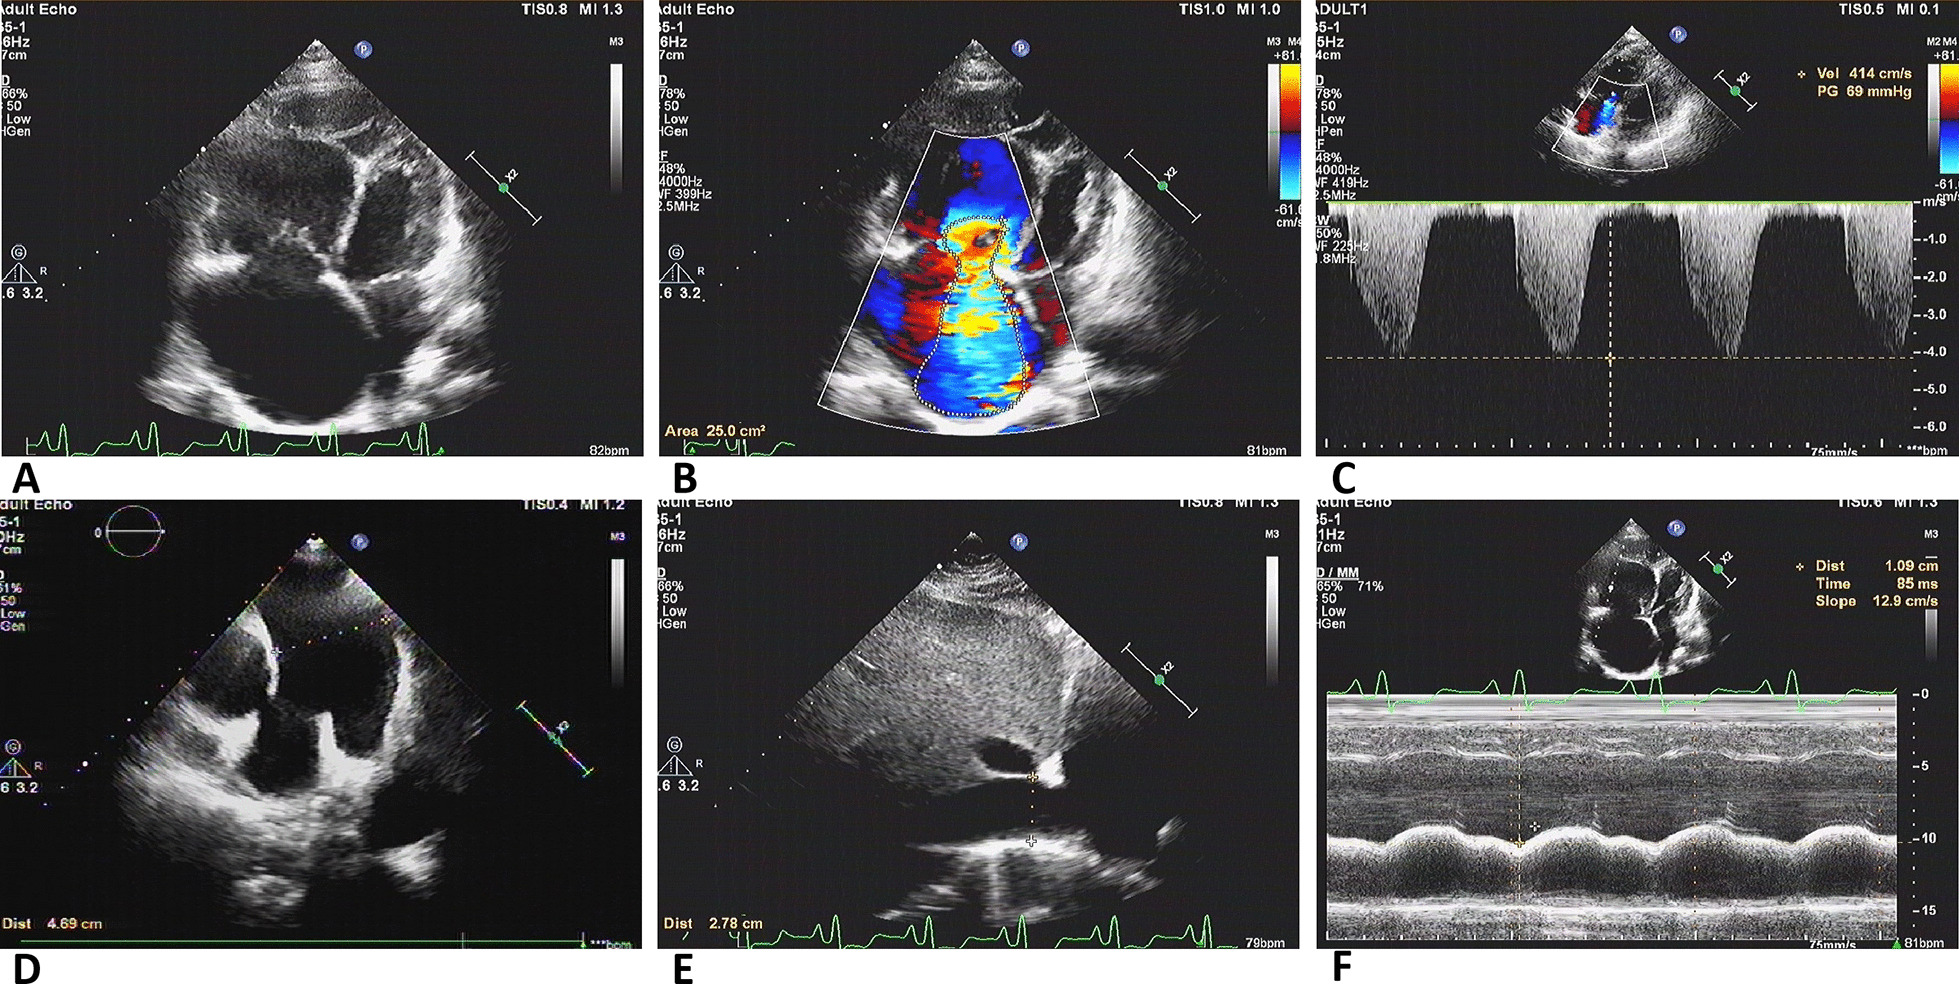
Echocardiography performed on patients with fibrosing mediastinitis complicated with pulmonary hypertension. a Echocardiography revealed an increase in right heart ratio. b Severe tricuspid regurgitation. c Tricuspid regurgitation with a Vel of 414 cm/sec and a PG of 69 mmHg. d Enlarged diameter of the main pulmonary artery (D_MPA_). e Enlarged diameter of the inferior vena cava (D_SVC_). f Decrease of approximately 10.9 mm in tricuspid annular plane systolic excursion (TAPSE)
